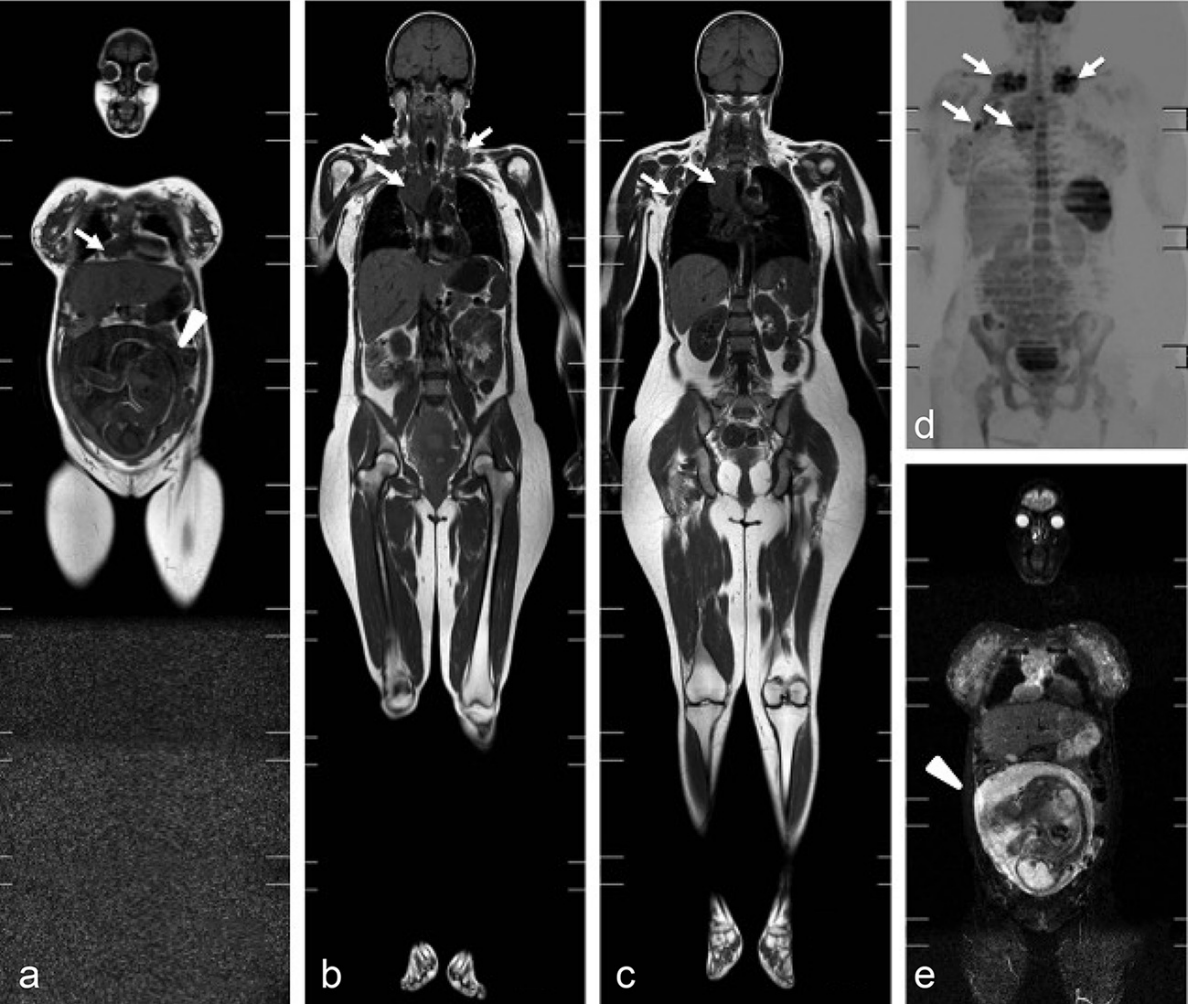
Pregnancy week 30 (a, e; arrowheads). Coronal *T*_1_ weighted (a–c), coronal maximum intensity projection diffusion-weighted (d) and coronal short tau inversion-recovery (e) whole-body MRI showing progression of the disease with right axillary involvement (c; arrows) and further enlargement of other lymph nodes involved (a–d; arrows).
